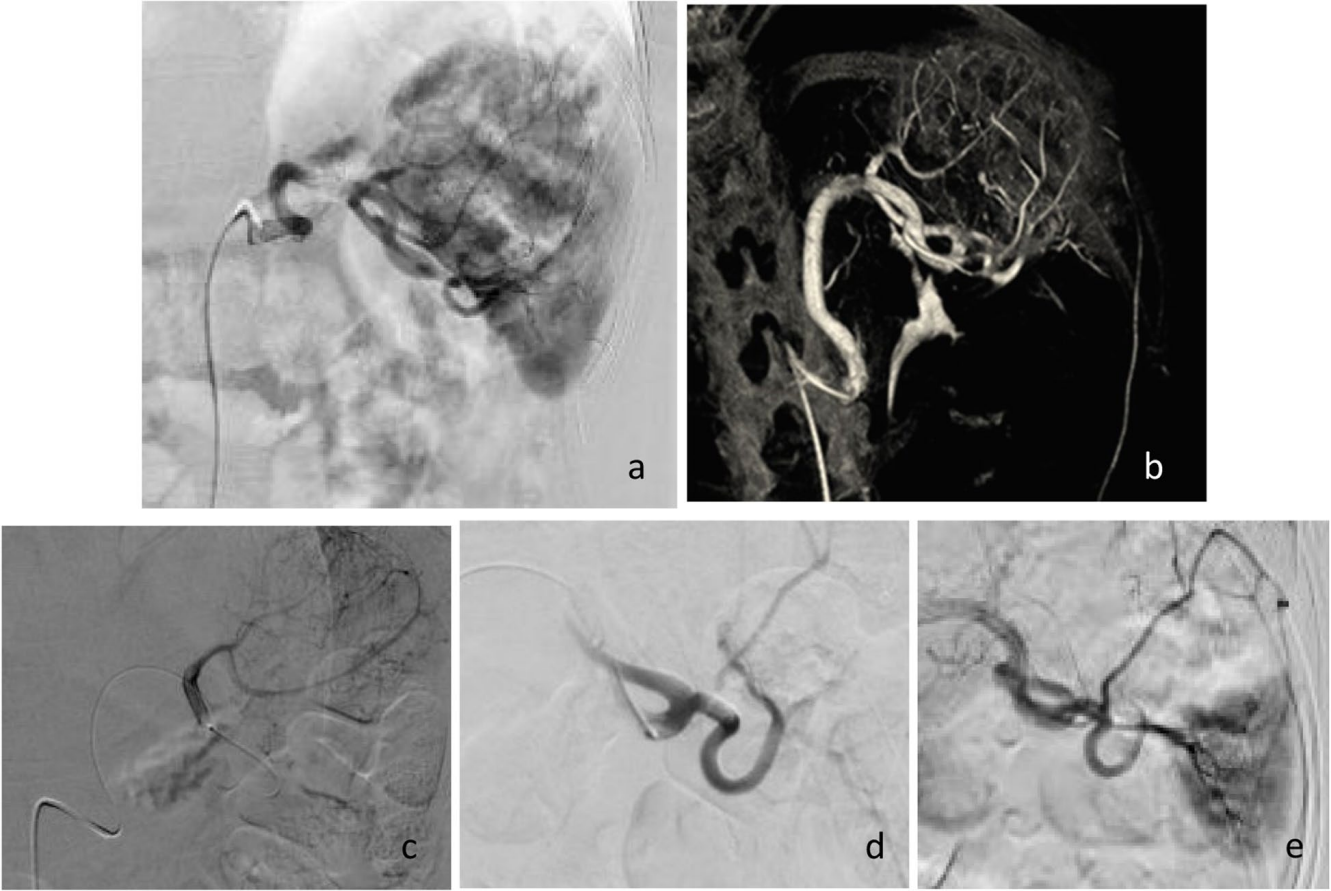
a DSA of the splenic artery and its branching pattern within the hemangioma. b CBCT provides 3D imaging of the hemangioma, its upper and lower feeding artery network, and its relation to close anatomical structures. Selective catheterization of splenic artery branches that feed the upper (c) and lower d half of the hemangioma. e Post-embolization image depicting the absence of blood supply to the hemangioma without affecting the remaining central and lower pole spleen parenchyma
